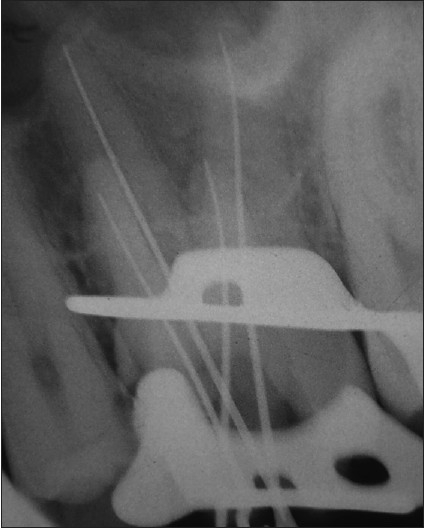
Working length radiograph of 26
